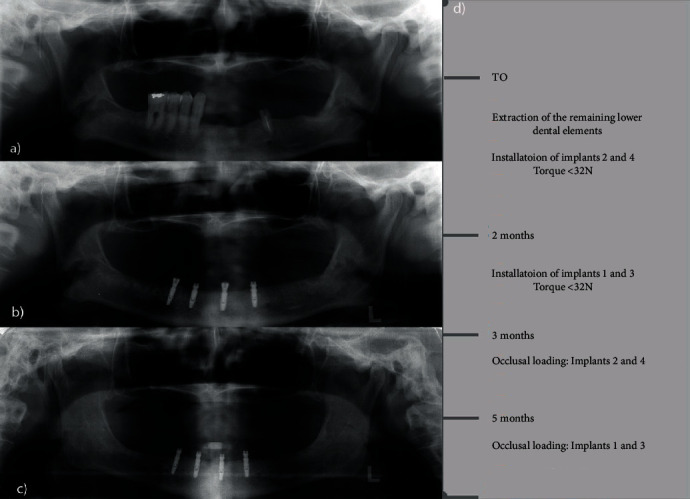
(a) Preoperative panoramic X-rays; (b) panoramic X-rays with installation of implants 2 and 4 (components installed) and implants 1 and 3 (healing caps); (c) final condition after loading all implants; (d) surgical timeline.
